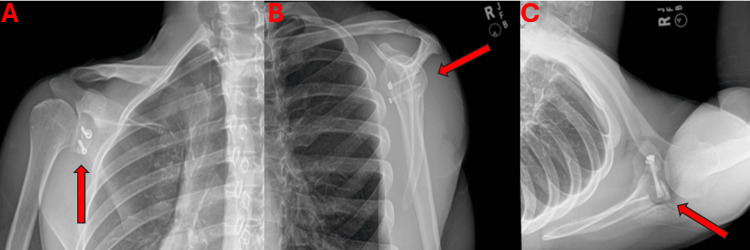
Radiographic images of the right shoulder obtained following traumatic injury demonstrating coracoid graft displacement and hardware failure after primary Latarjet procedure. Pre-revision radiographic views of the right shoulder demonstrating posterior displacement of the coracoid graft with hardware failure, including a bent proximal screw and posterior migration of the distal screw, shown in anterior (A), posterior (B), and axial (C) orientations.
